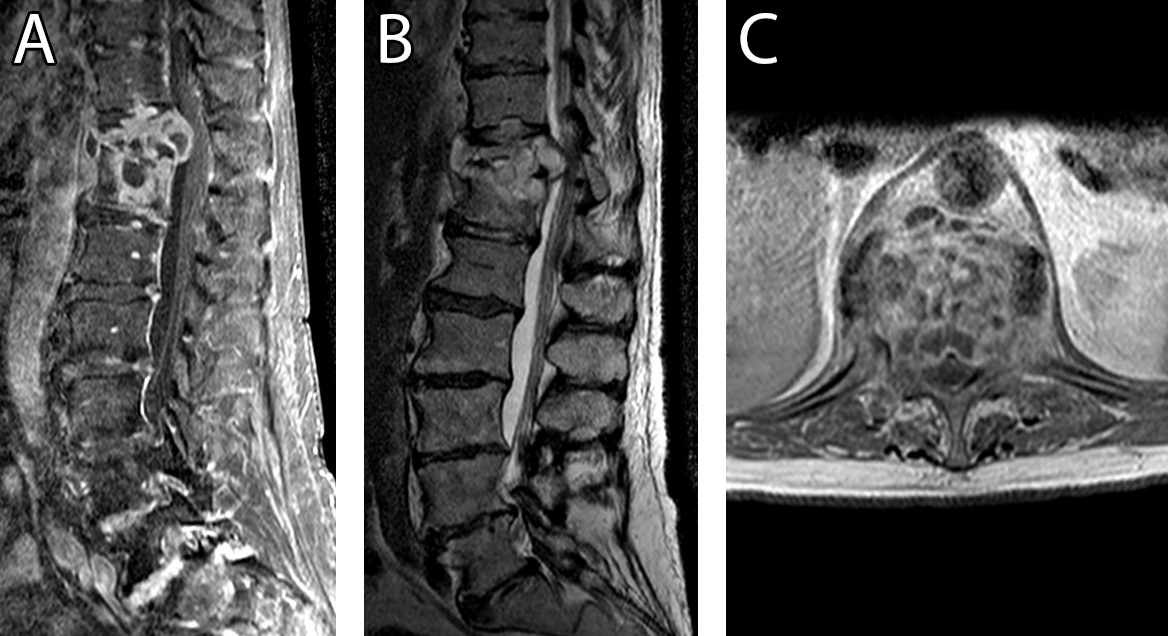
Preoperative MRI A) Preoperative sagittal T1-weighted MRI with contrast, B) sagittal T2-weighted MRI demonstrating T11-12 osteomyelitis with intervening discitis and paraspinal enhancement, and C) axial T1-weighted MRI with contrast demonstrating epidural phlegmon resulting in neural compression.
